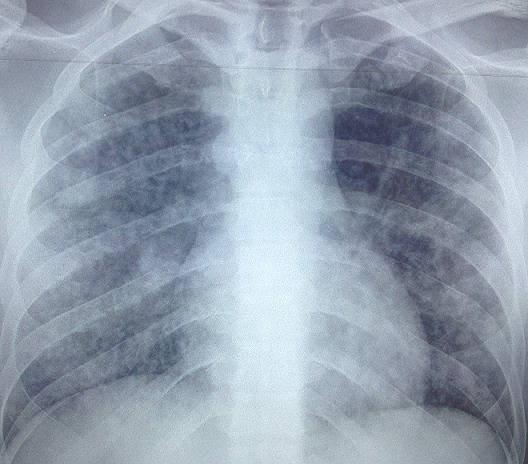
Radiographie du thorax de face qui montre la présence d'opacités alvéolo-interstitielles bilatérales et diffuses aux deux champs pulmonaires
